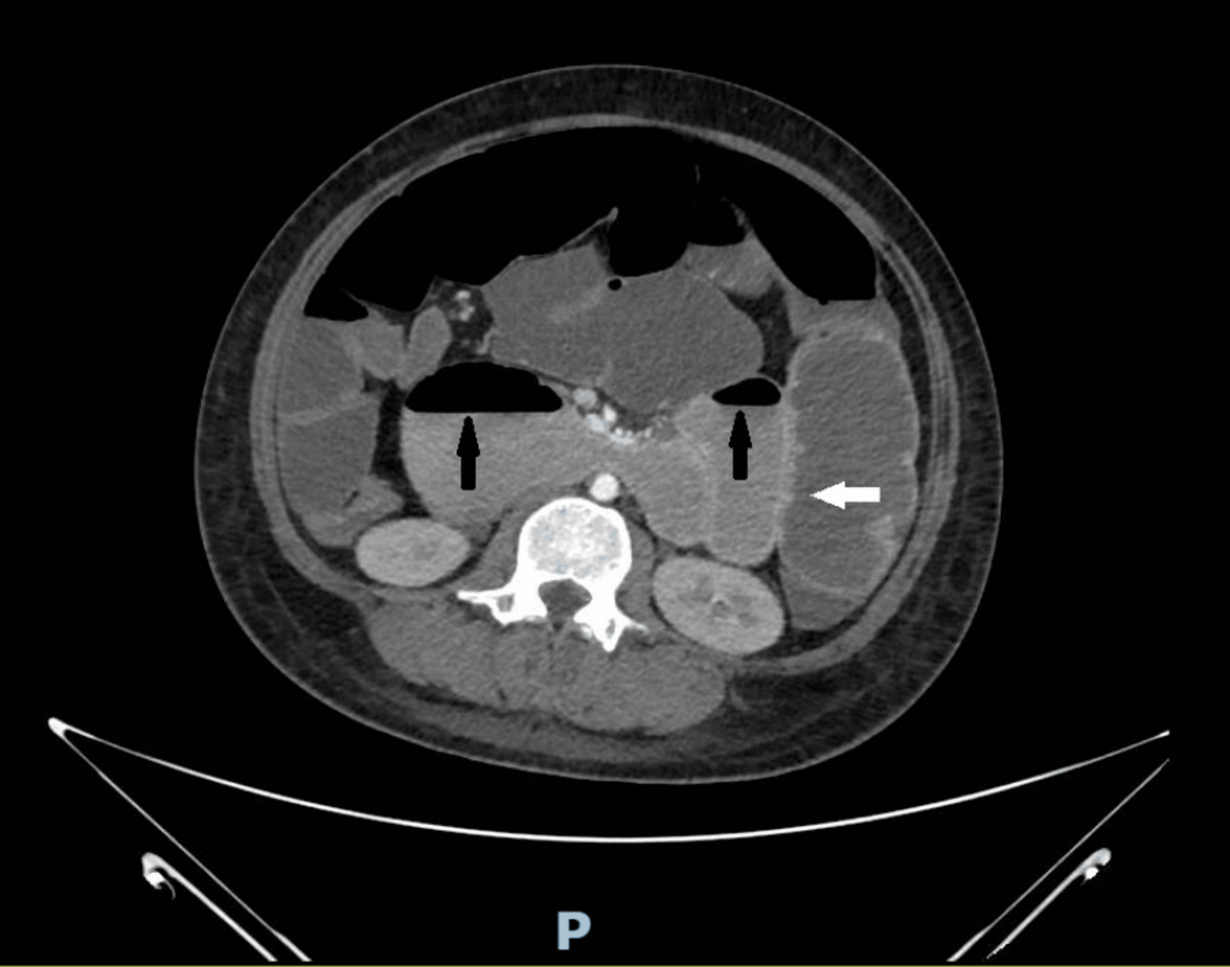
CECT of the abdomen (transverse section) showing air-fluid levels (black arrows) and bowel wall thickening (white arrow) CECT, contrast-enhanced computed tomography
